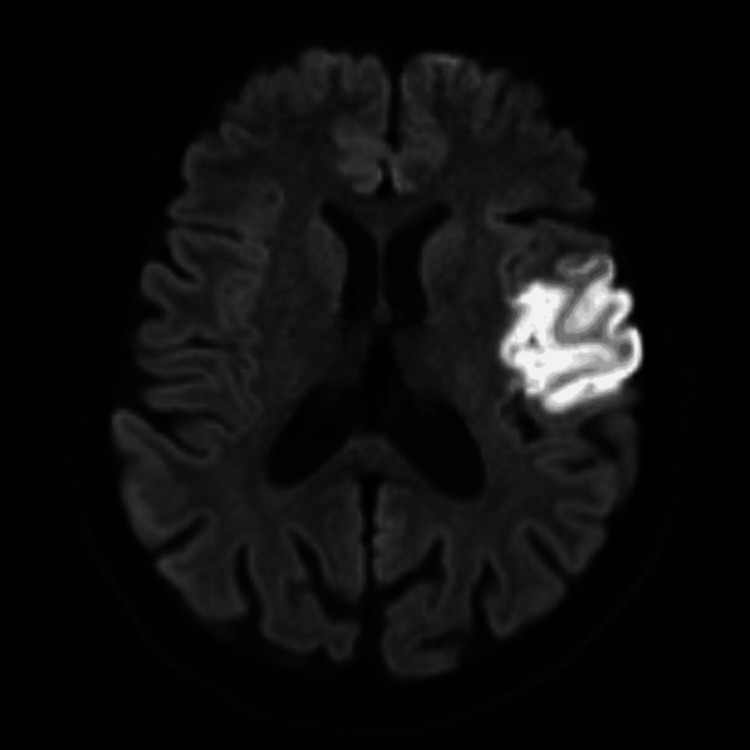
Brain magnetic resonance imaging showing an acute ischemic infarct in the left middle cerebral artery territory, affecting the insula and the frontoparietal lobe and extending to the pre- and post-central gyri.
